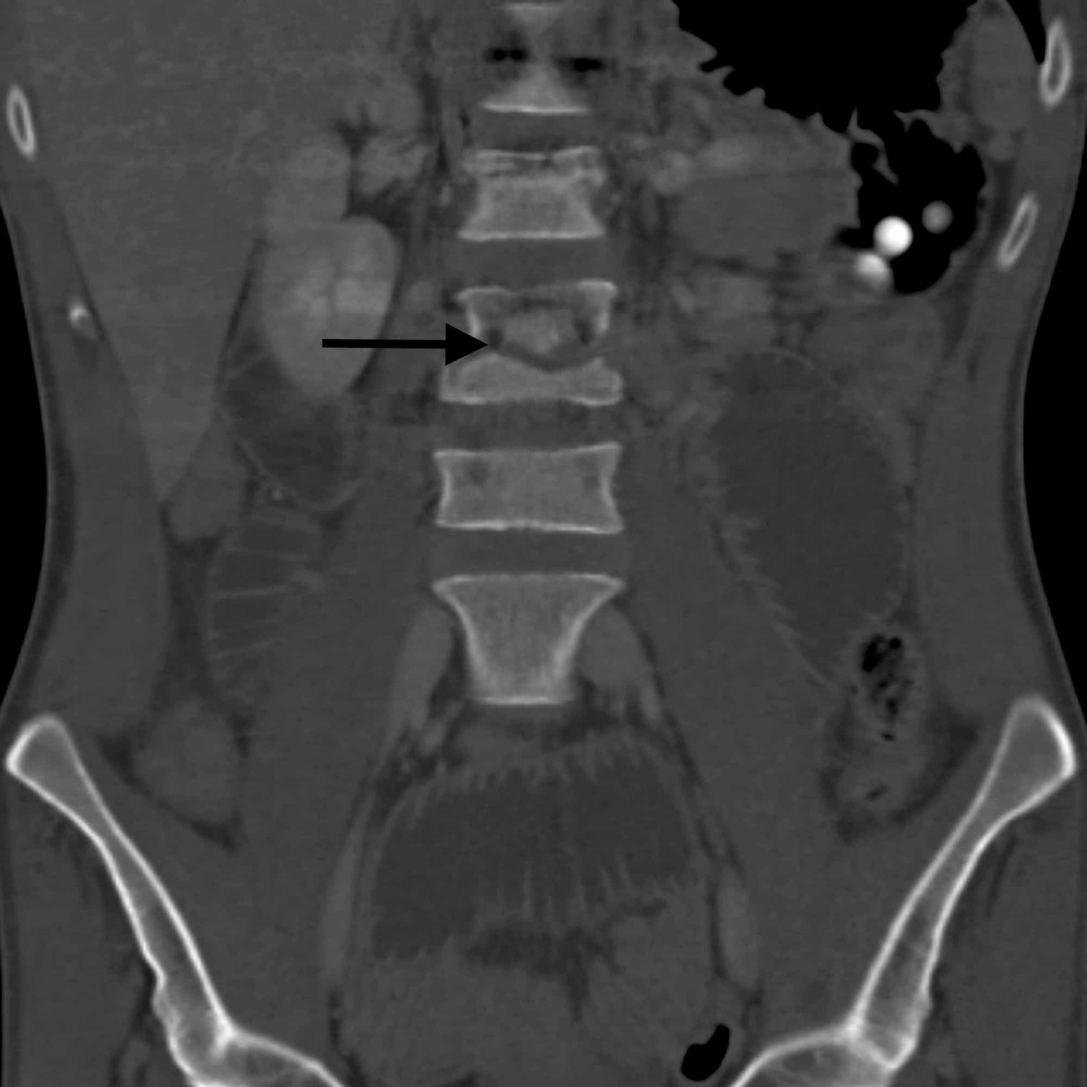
Coronal CT showing a ventral defect at L3.
